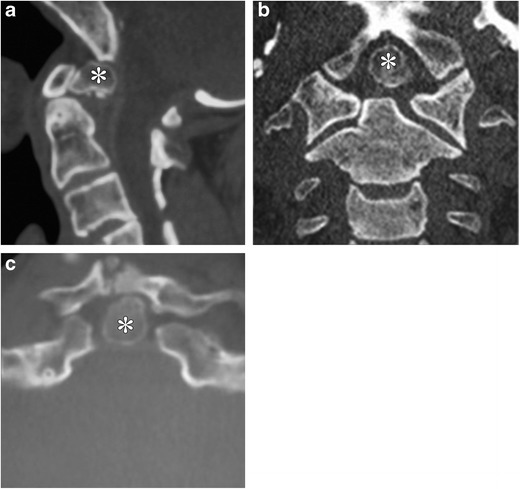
Dystopic os odontoideum in a 19 year old: Sagittal (*a*), coronal (*b*) and axial (*c*) images from a non-contrast CT in a 19 year old demonstrate a well-corticated ossific density along the superior margin of the dens (marked by *asterisks* on images *a*, *b* and *c*). The dens itself is relatively small with a foreshortened base (*block arrow* on *b*). Note that the os odontoideum is located somewhat posterior to the C2 body and is therefore not in its expected anatomic location
